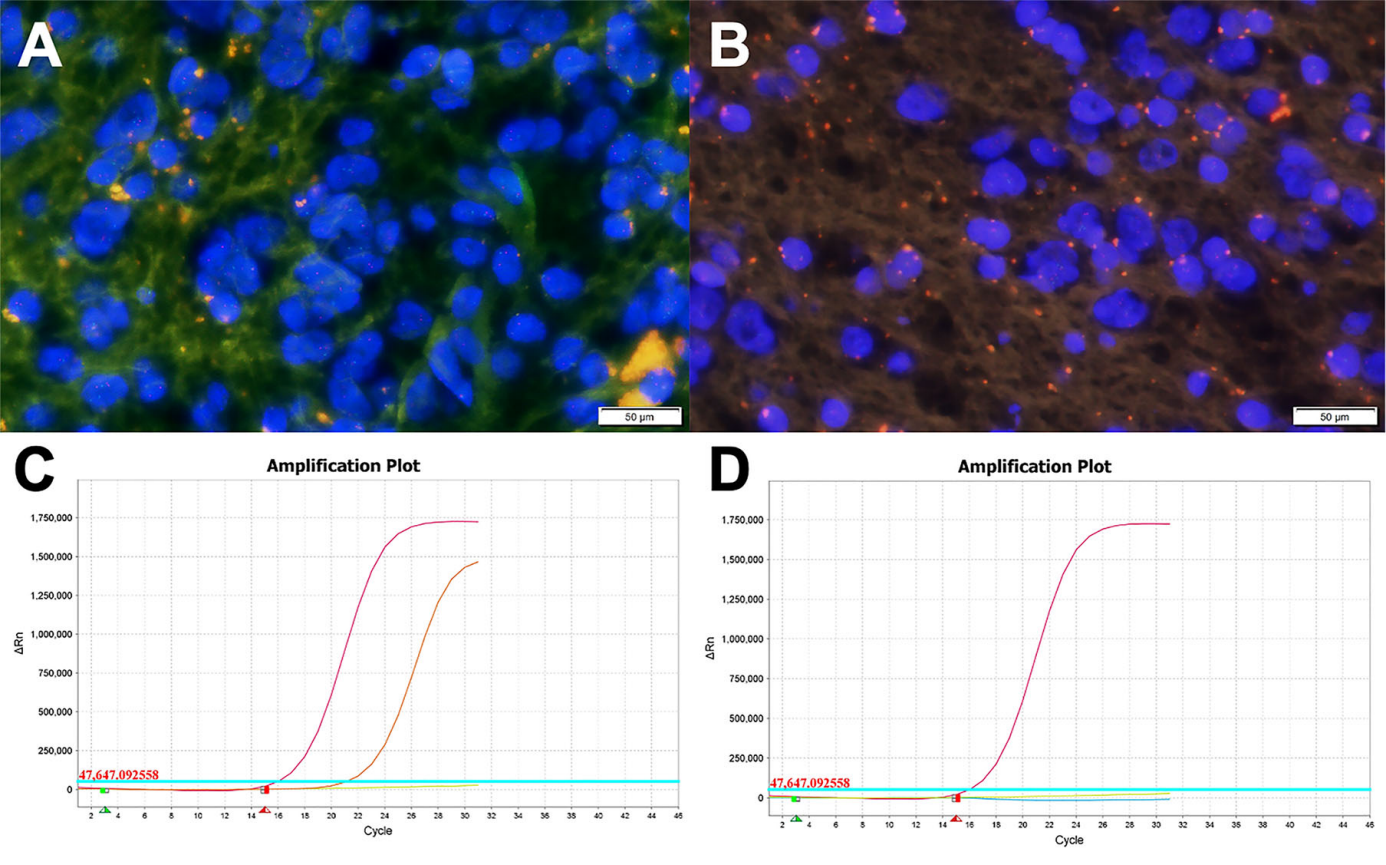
Molecular examination images of PLNTY. (A, B) FISH analysis for 1p/19q showing absence of chromosome 1p/19q deletion (Case c). (C) The BRAF V600E gene mutation was detected by the Real-Time PCR technique. The orange curve, the red curve, the green line, and the blue line represented the tested sample, the positive control, the negative control, and the threshold line respectively. The FAM signal, as seen in Case c, had an obvious amplification curve, and the CT value is less than 28, which means the BRAF V600E mutation is positive. (D) The BRAF V600E gene mutation of Case a (the blue line representing the tested sample). The test result was negative for the BRAF V600E gene mutation as the FAM signal had no amplification curve.
